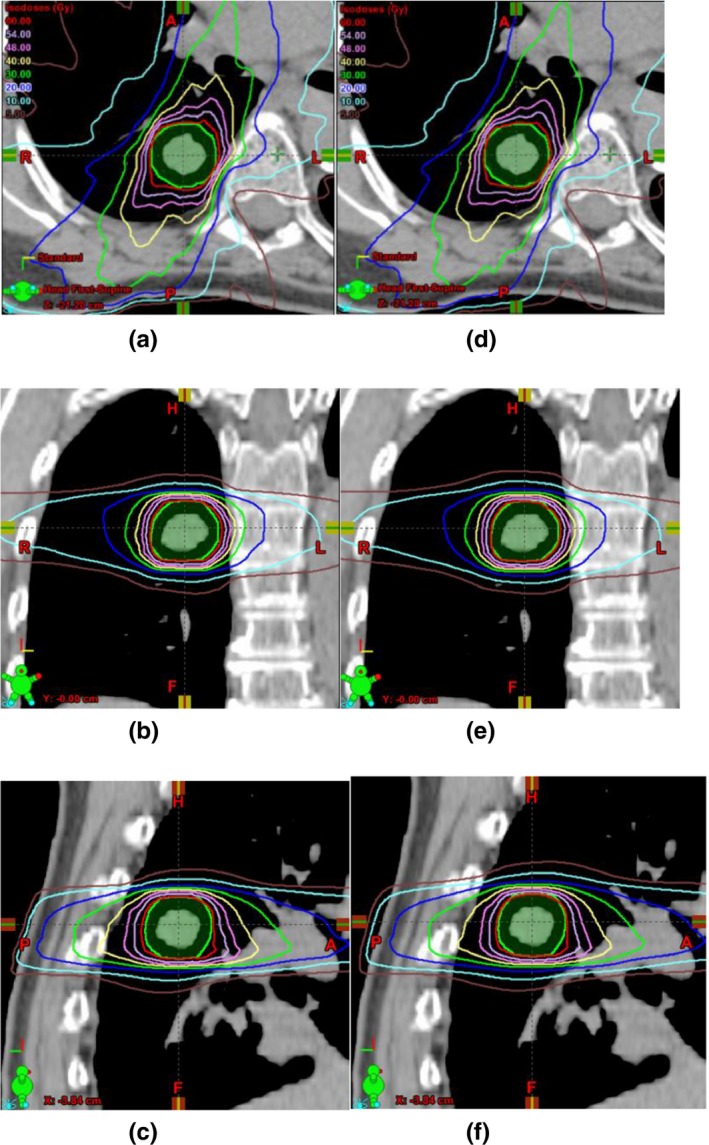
Comparison of isodose distributions for the 3D (a), (b), and (c) and 4D (d), (e), and (f) VMAT plans are shown in the left and right panels respectively. Both plans were generated on the 50% CT image for a lung case with target motion amplitude of 1.6 cm for patient #1.
